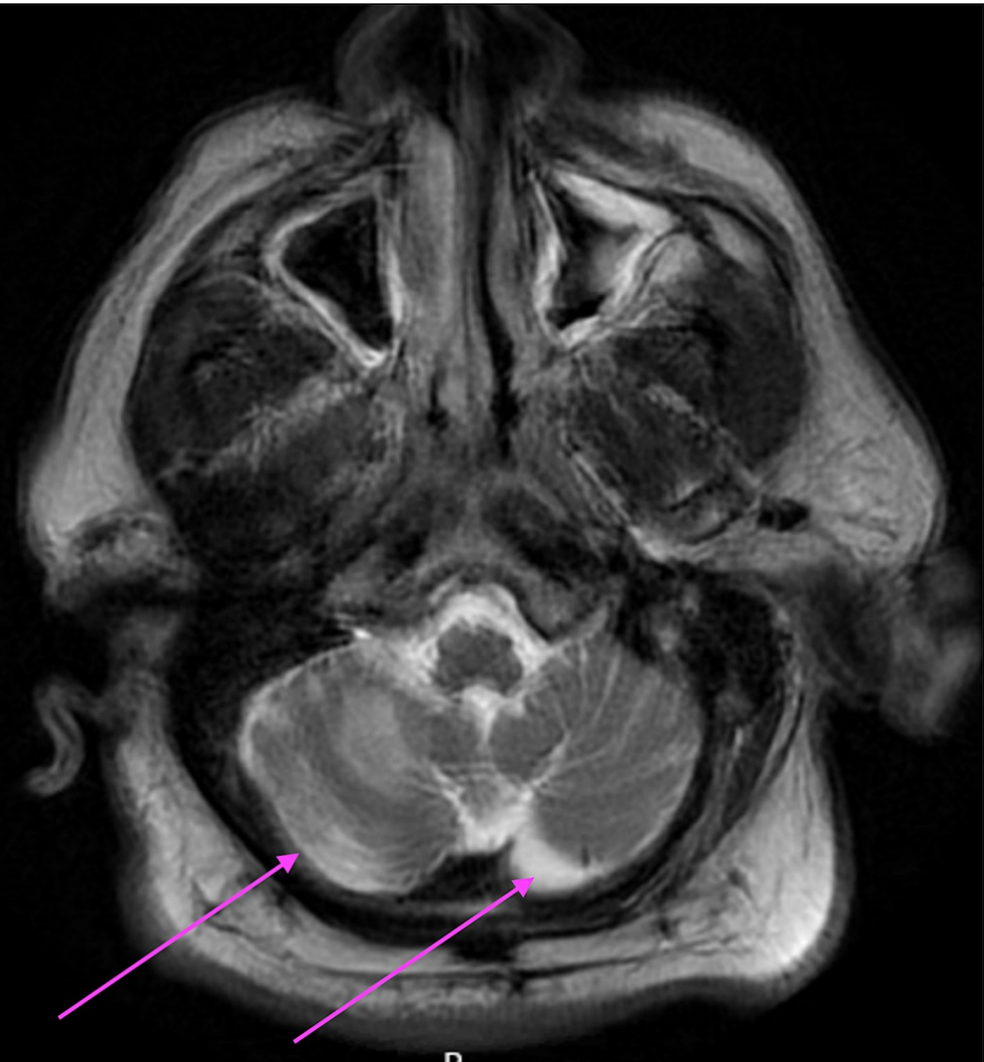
MRI brain showing bilateral cerebellar white matter signal abnormalities in COVID-19 cerebellitis
